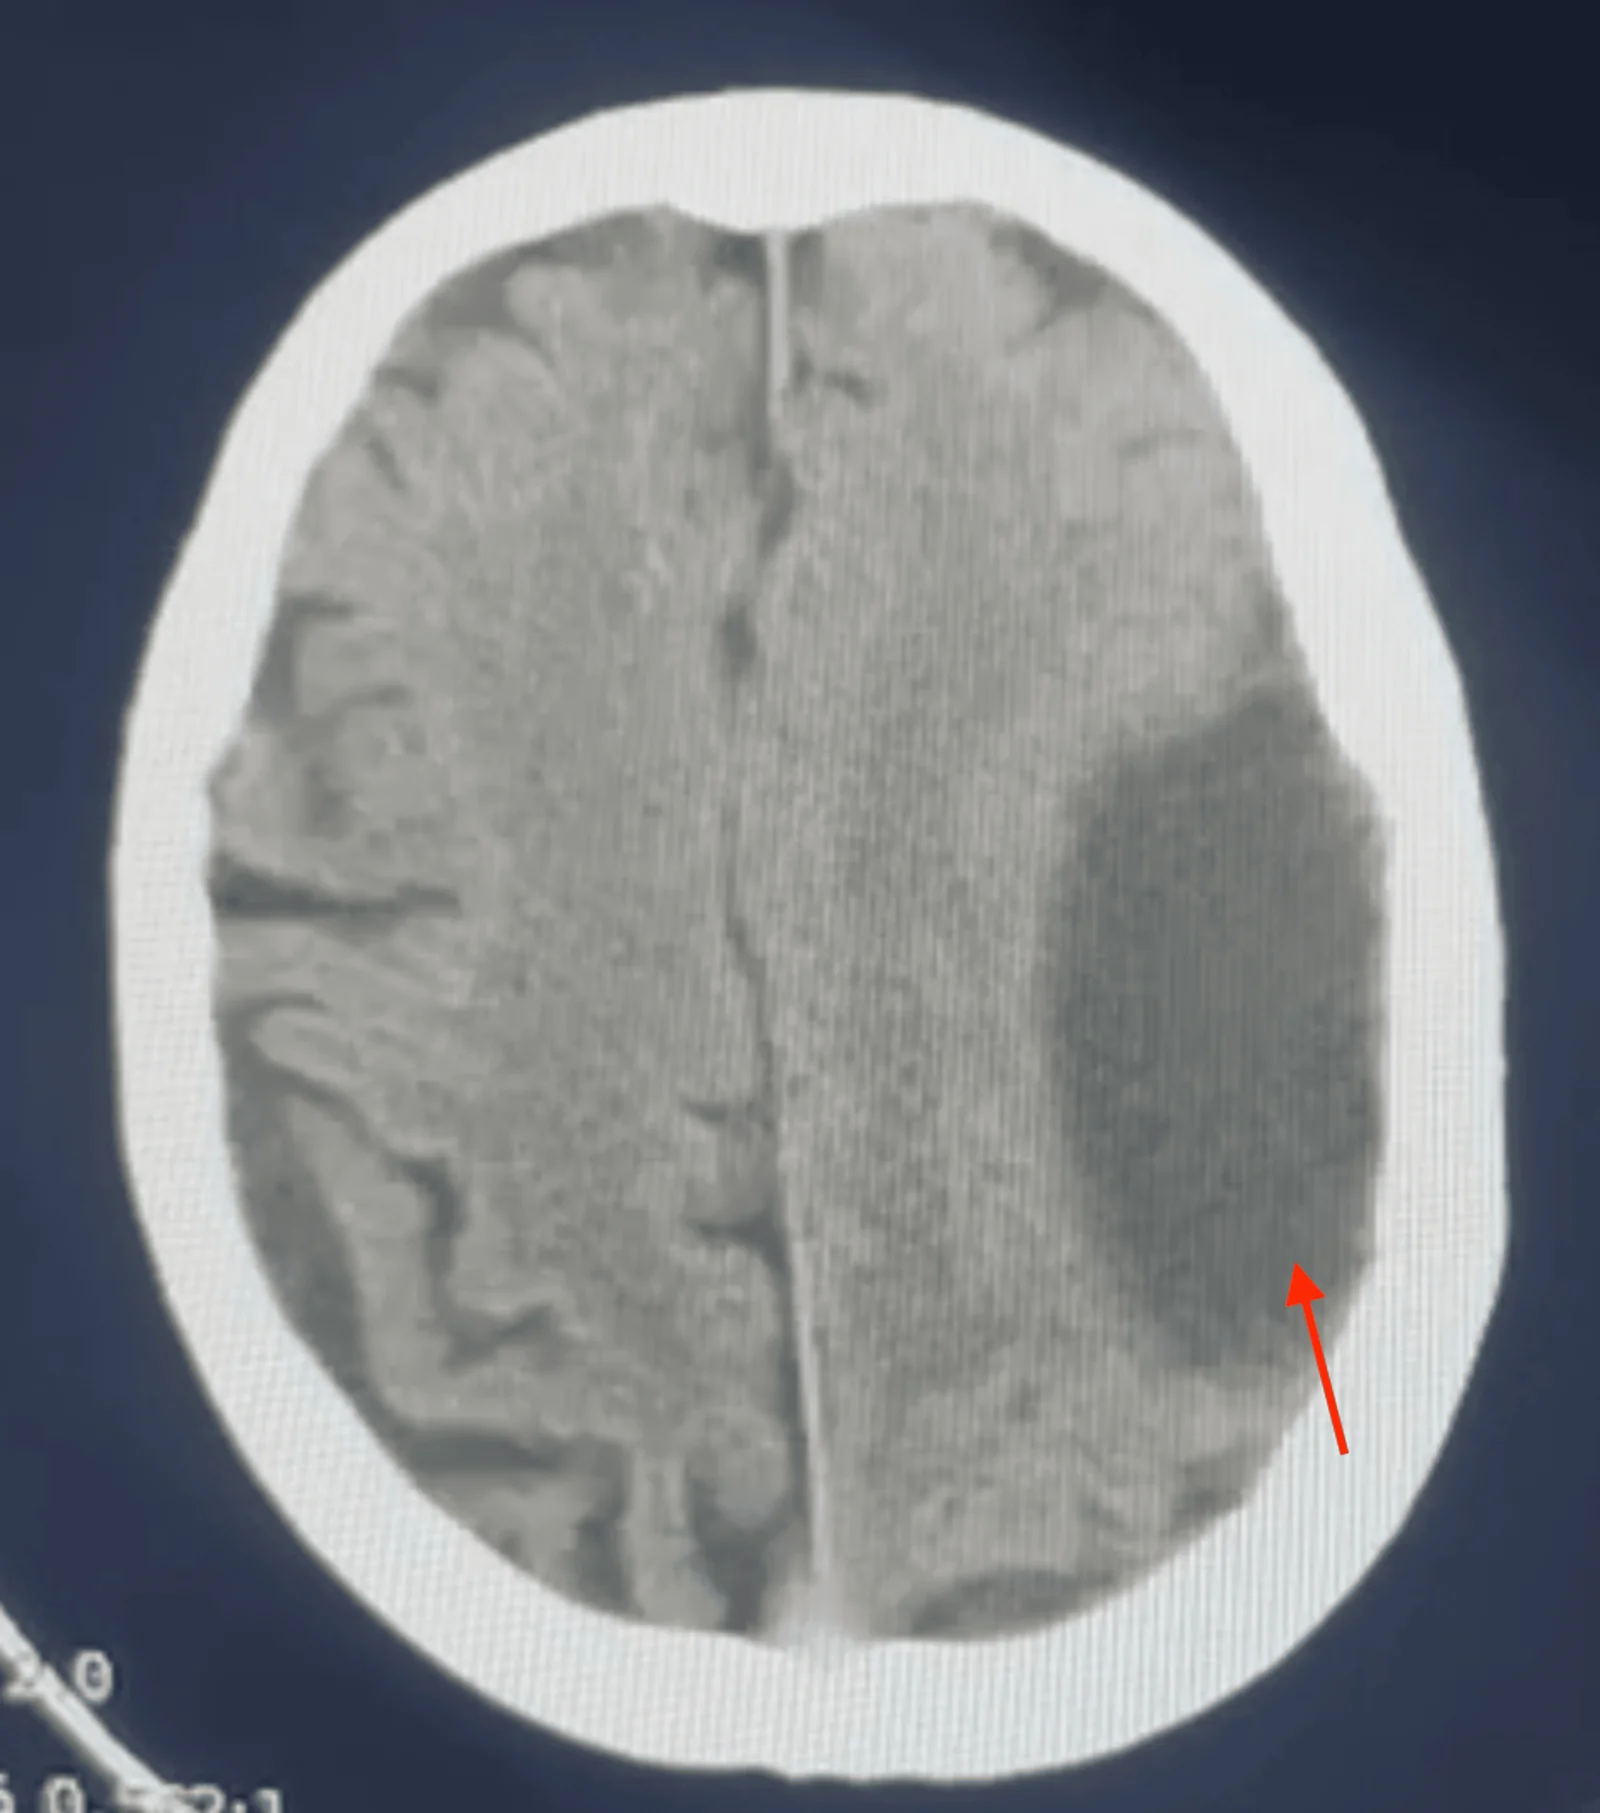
Non-contrast axial cranial CT scan showing a hypodense lesion in the left fronto-parietal region (red arrow).
